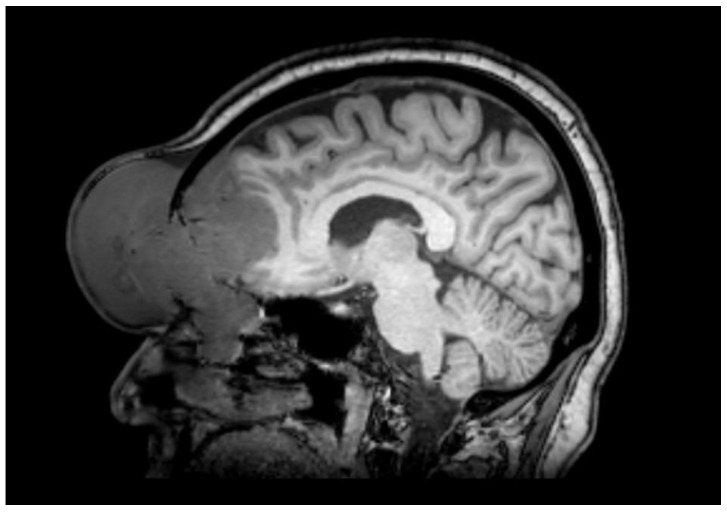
Magnetic resonance imaging (MRI): uniformly enhanced mass in the right frontal sinus with anterior invasion of subcutaneous soft tissues, posterior intracranial involvement, and erosion of the anterior ethmoidal roof and lamina papyracea.
